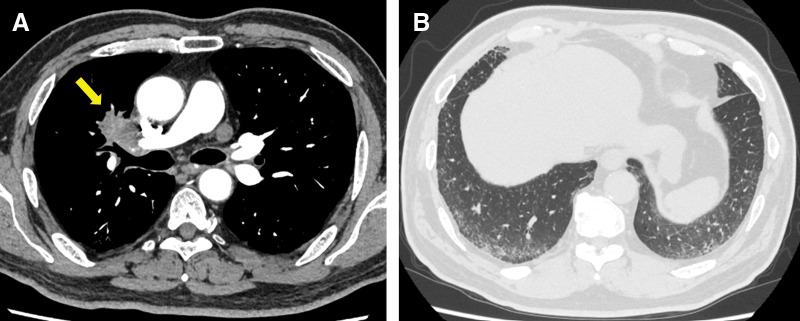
Preoperative CT findings. (A) Contrast-enhanced CT revealing a tumor measuring 5.0 cm in diameter in the right upper hilum (yellow arrow). (B) Interstitial pneumonia was observed at the bases of the bilateral lungs.
